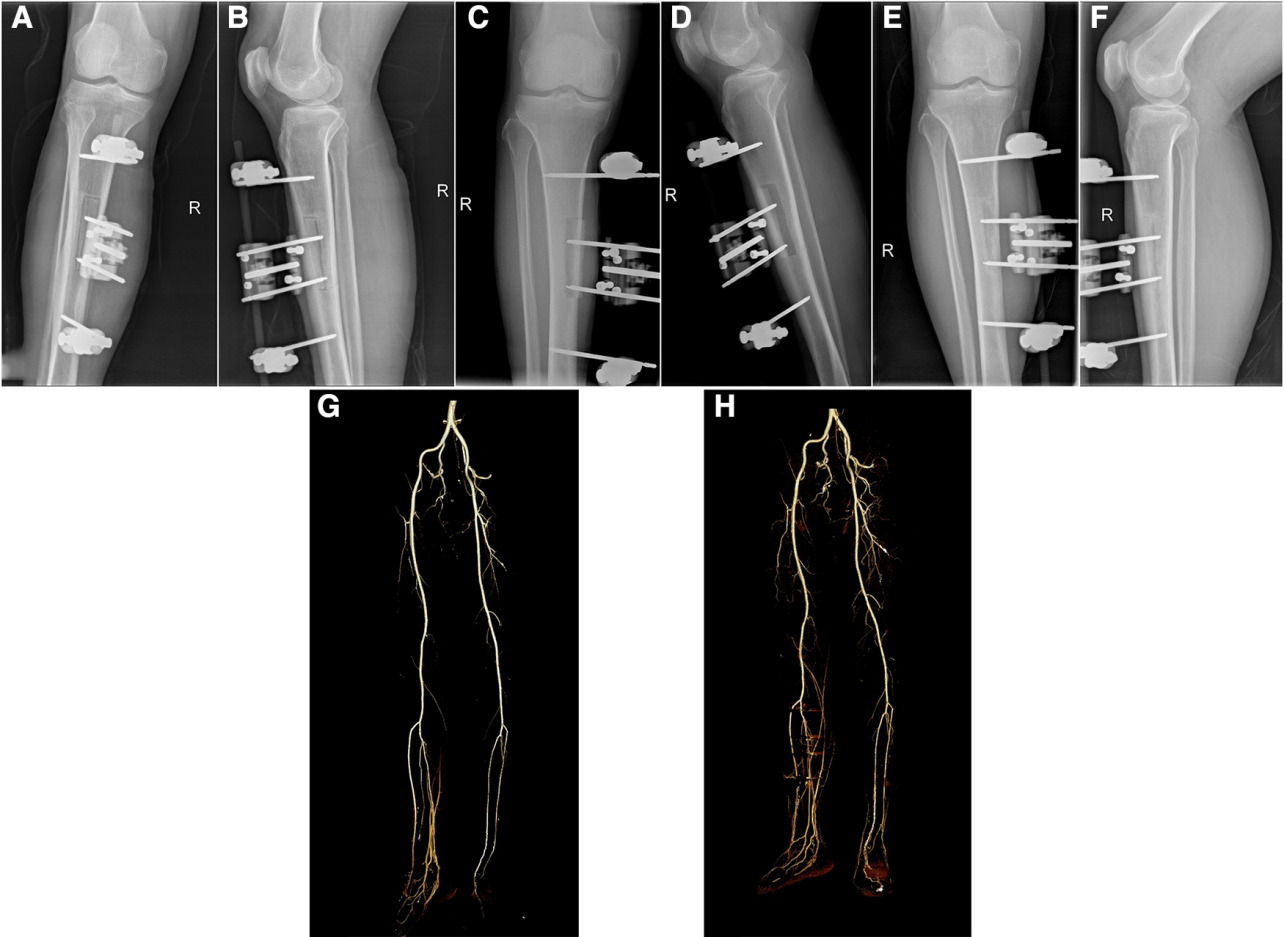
Imaging evaluation of typical case 1. (A,B) Immediate x-ray image after TTT surgery; (C,D) X-ray image at 12 days after bone cortex transport; (E,F) X-ray image at 2 months after TTT surgery; (G) Pre-operative image of CTA; (H) Image of CTA at 2 months after TTT surgery.
